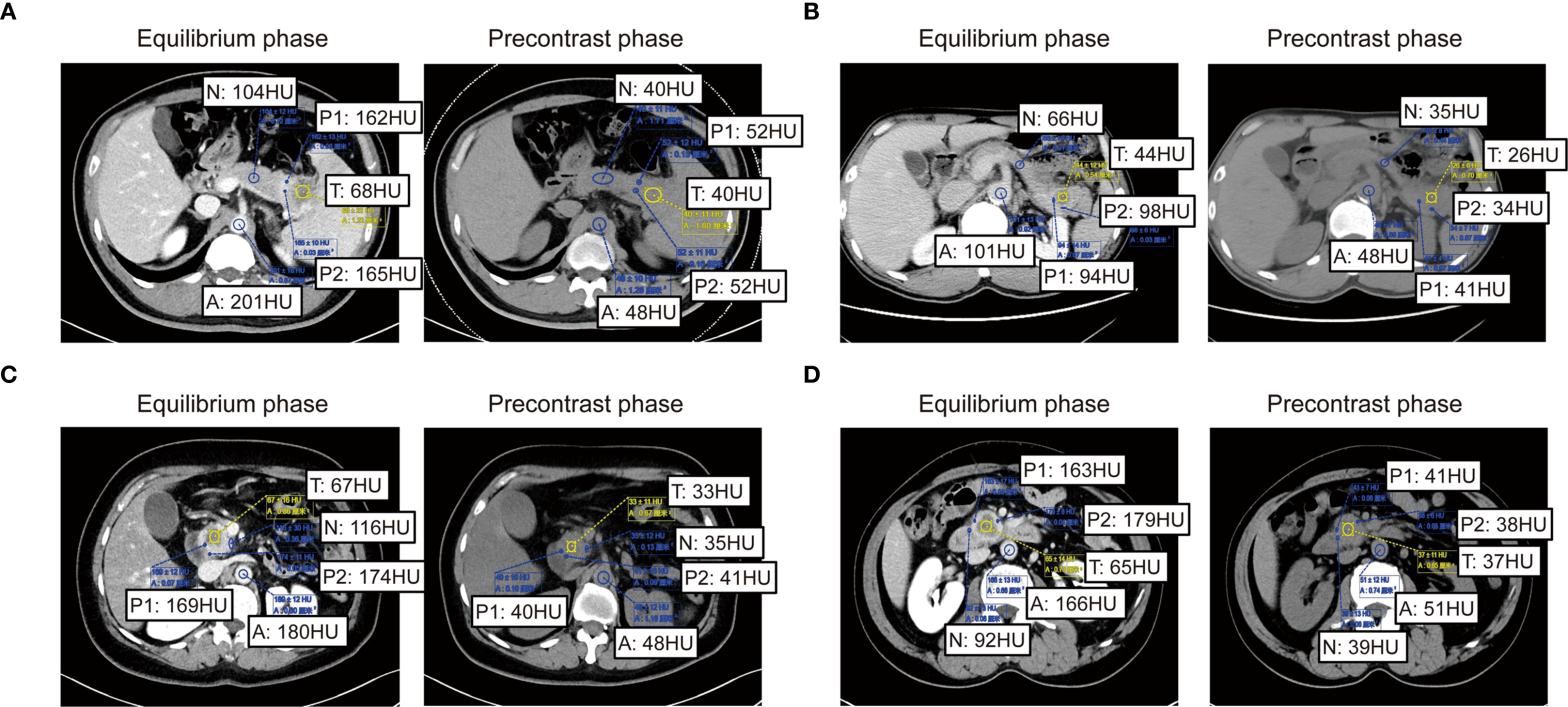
Imaging-based quantification of PC fibrosis metrics. (A–D) Quantitative presentation and comparison of HU values across tumor core, tumor periphery, normal pancreatic parenchyma HU values, and abdominal aorta HU values in identical patients during pre-contrast and equilibrium phases of contrast-enhanced CT.
